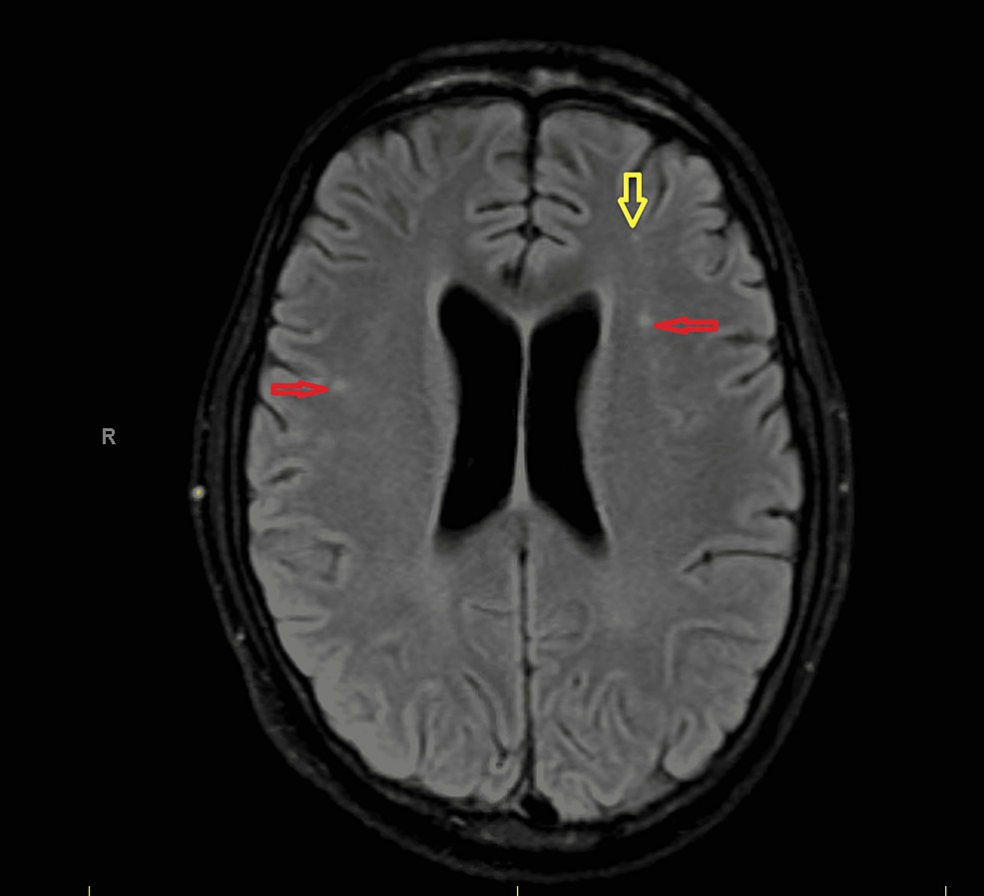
MRI brain without contrast: red arrows point to the small areas of high signal intensity on the FLAIR in the along the centrum semiovale; yellow arrows point to the high signal intensity on the FLAIR in the periventricular and subcortical white matter.
